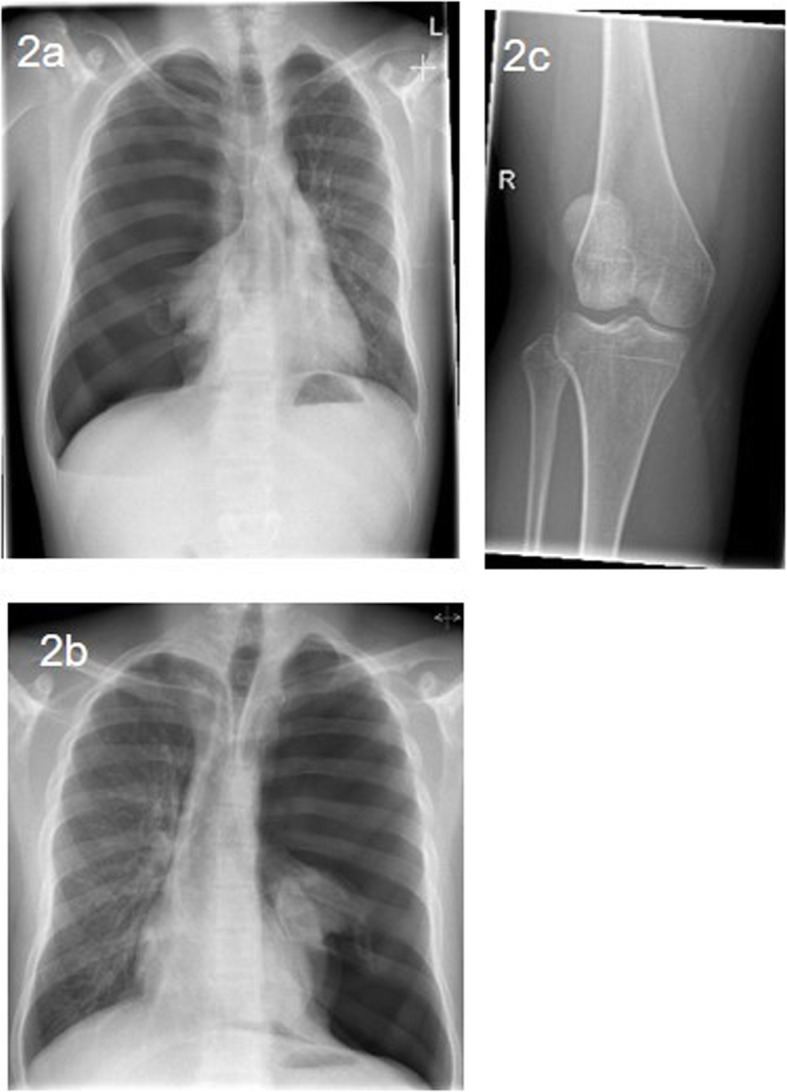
a Patient 1: chest x-ray, first tension seropneumothorax right side with mediastinal shift, 15y; b Patient 1: chest X-ray, tension pneumothorax left side with mediastinal shift, 16y; c Patient 1: X-ray right knee because of patellar luxation, no osteopenia, 20y
